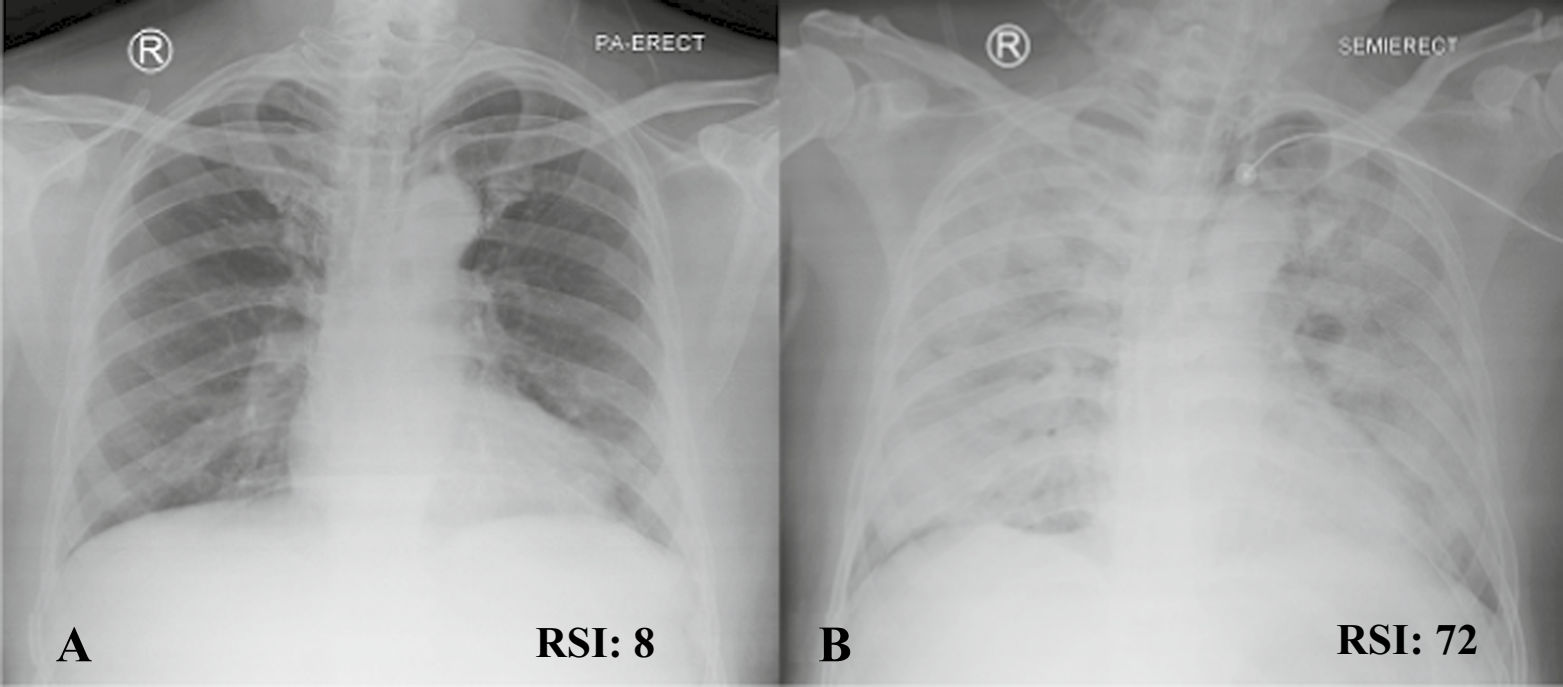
Serial radiologic severity index in COVID-19 pneumonia patient. A Initial chest X-ray RSI at 22 March 2020; B serial chest X-ray at 29 March 2020. An increase in the RSI value can be seen from an increase in the area and density of the lesion on the first and second chest X-rays
